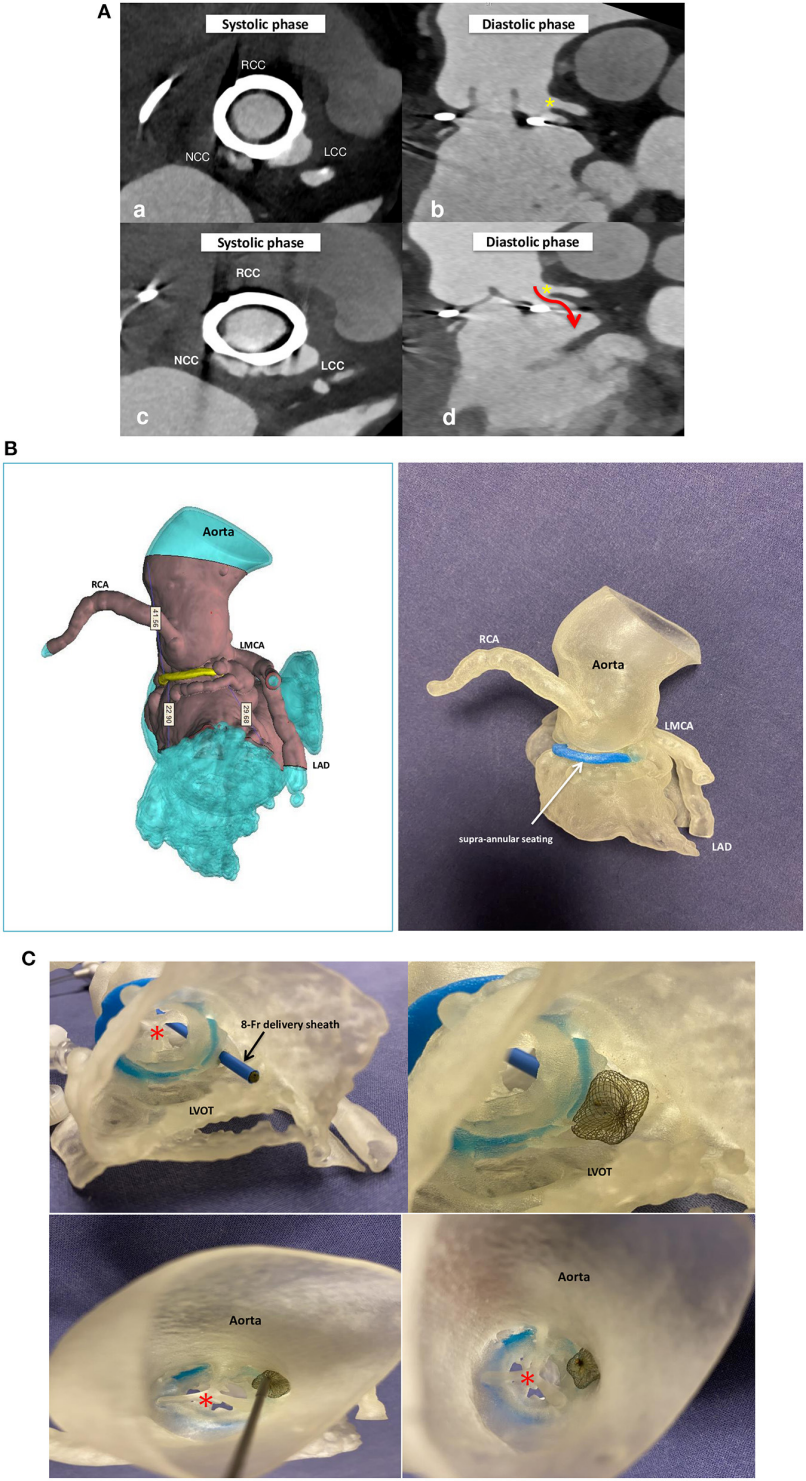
Multidetector computed tomography angiography (MDCTA) scans (A) with acquired frames in systolic (a,c) and diastolic phase (b,d) confirming the presence of the paravalvular pseudoaneurysm fistula located at the left coronary cusp in close proximity of the left main coronary artery (yellow star) and protruding in diastole into the left ventricle outflow tract (red arrow). Silicone printed 3D heart model made on the basis of MDCTA scan reconstruction (B). Silicone 3D model seen from the left ventricle outflow tract (upper panels) and from the ascending aorta (bottom panels) and used to mimic the implantation of a self-expanding double-disc 5-mm square twist Occlutech PLD, with a distal left ventricle disc of 10 mm and a proximal aortic disc of 8.5 mm. Note that the device does not impinge on the bioprosthetic aortic valve (red star). RCC, right coronary cusp; LCC, left coronary cusp; NCC, non-coronary cusp; RCA, right coronary artery; LMCA, left main coronary artery; LAD, left anterior descending; LVOT, left ventricle outflow tract.
